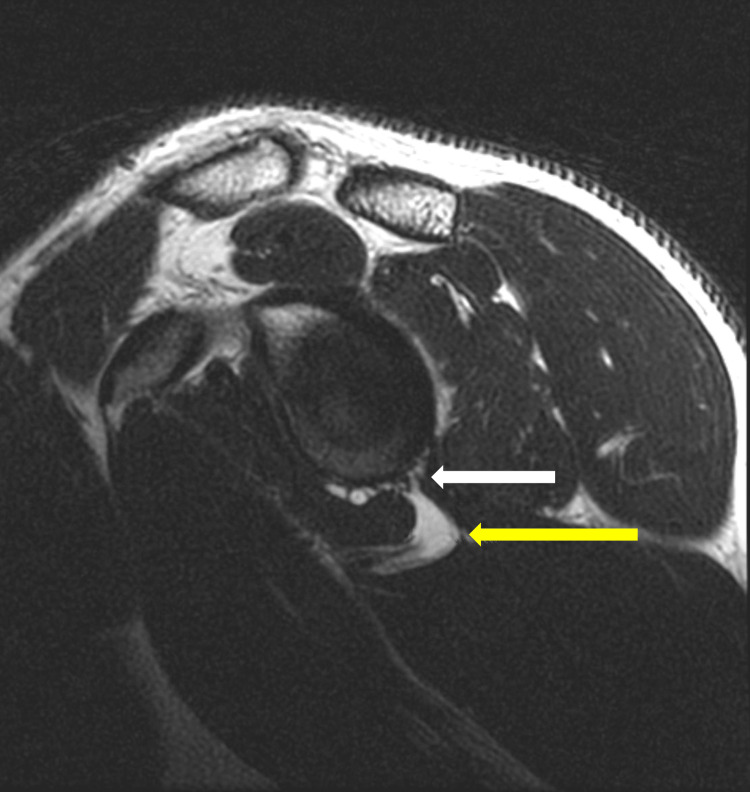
Parasagittal T1-weighted MRI demonstrating a paralabral cyst inferior to the glenoid, extending into the quadrilateral space and abutting the neurovascular bundle The yellow arrow demonstrates the cyst. The white arrow demonstrates the neurovascular bundle
